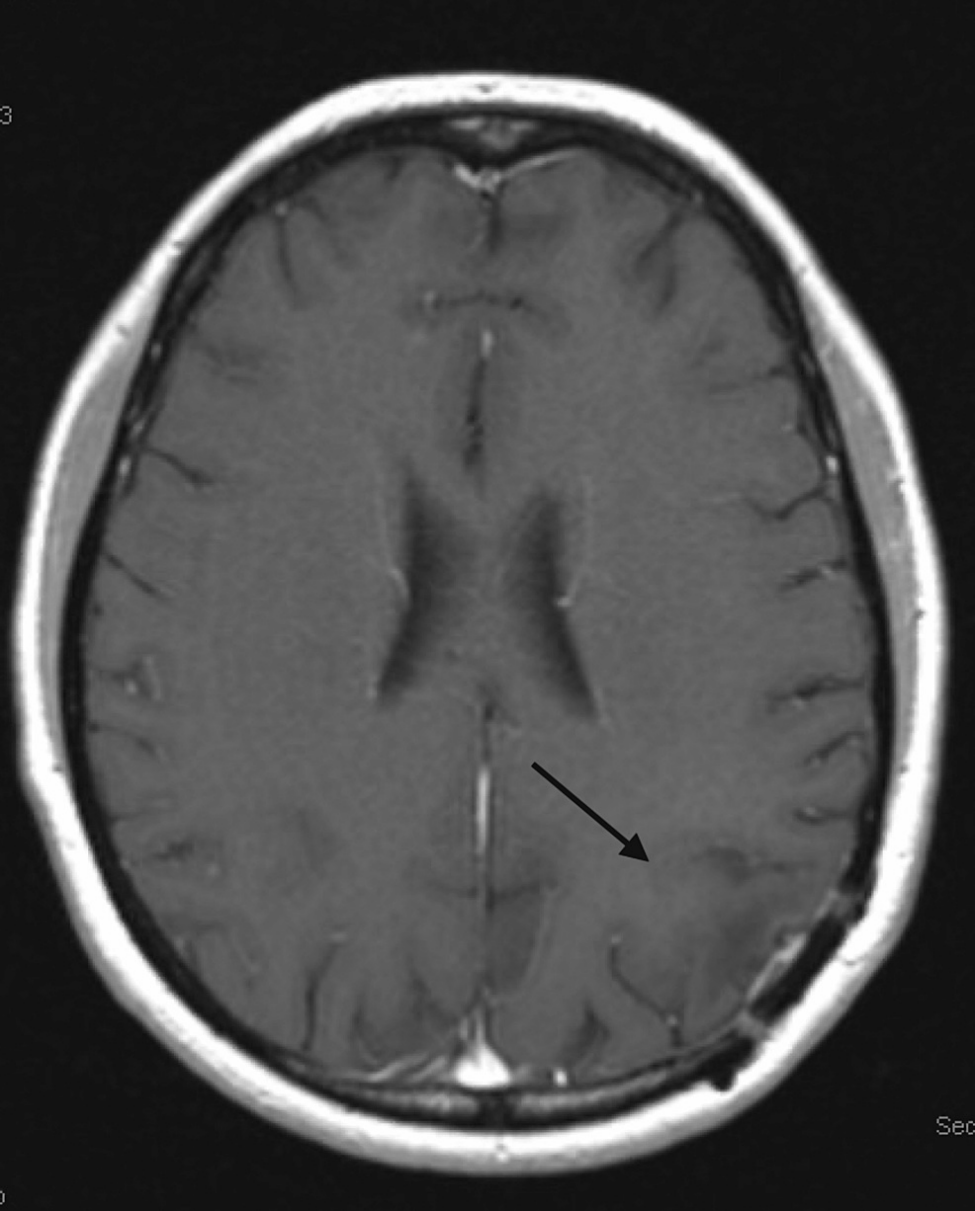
MRI of the brain showing improvement in the previously described enhancement of the resection cavity. MRI: magnetic resonance imaging
